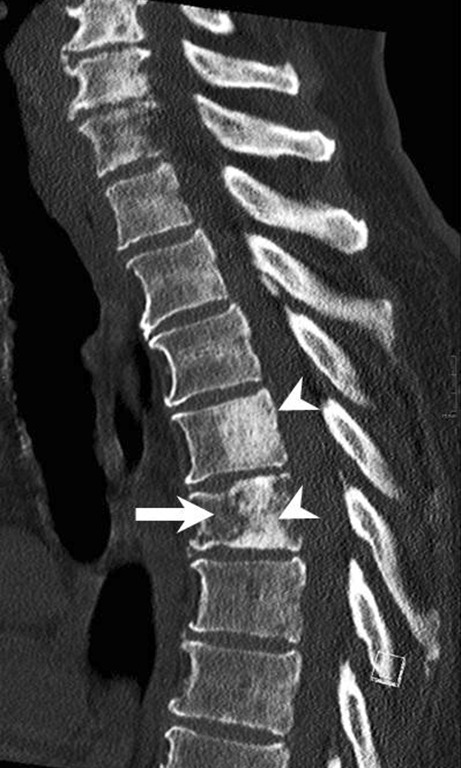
Sagittal reconstructions of computed tomography images of the thoracic spine in bone window setting showed increased density of large portions of the vertebral bodies of Th4 and Th5 (*arrowheads*). The vertebral body of Th5 showed a lytic area in its ventral portion and a compression fracture (*arrow*)
